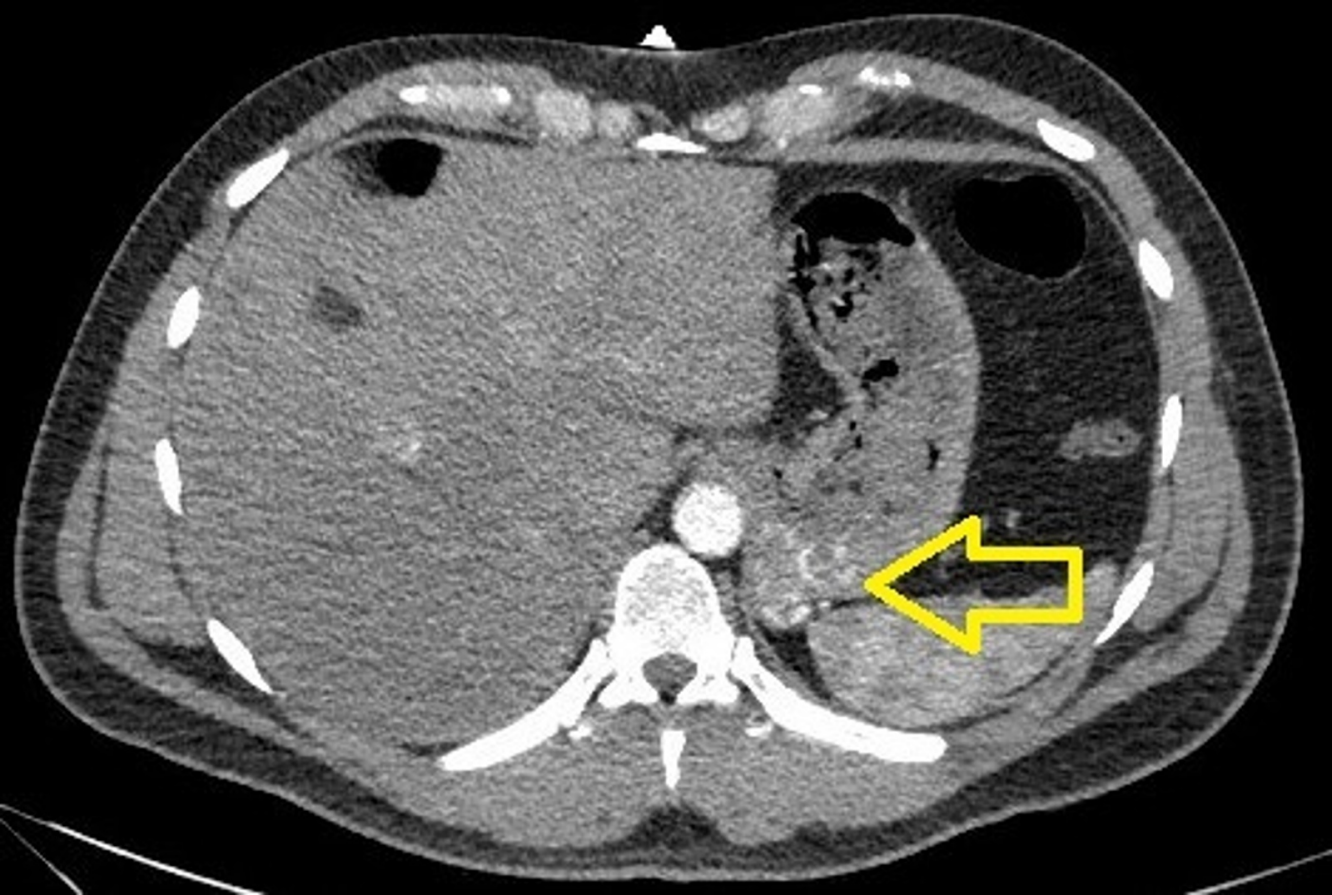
CTA of the abdomen showing a small tangle of enlarged tortuous blood vessels along the posterior cardia of the stomach.
